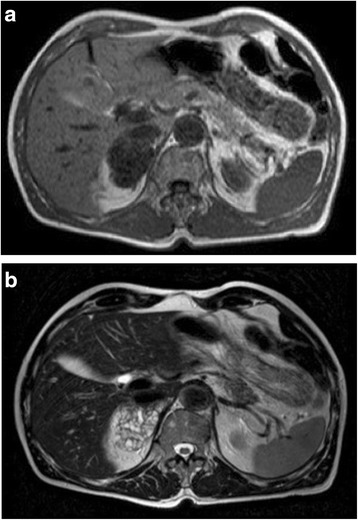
a, b Magnetic resonance imaging T1-weighted and T2-weighted images of right adrenal incidentaloma
